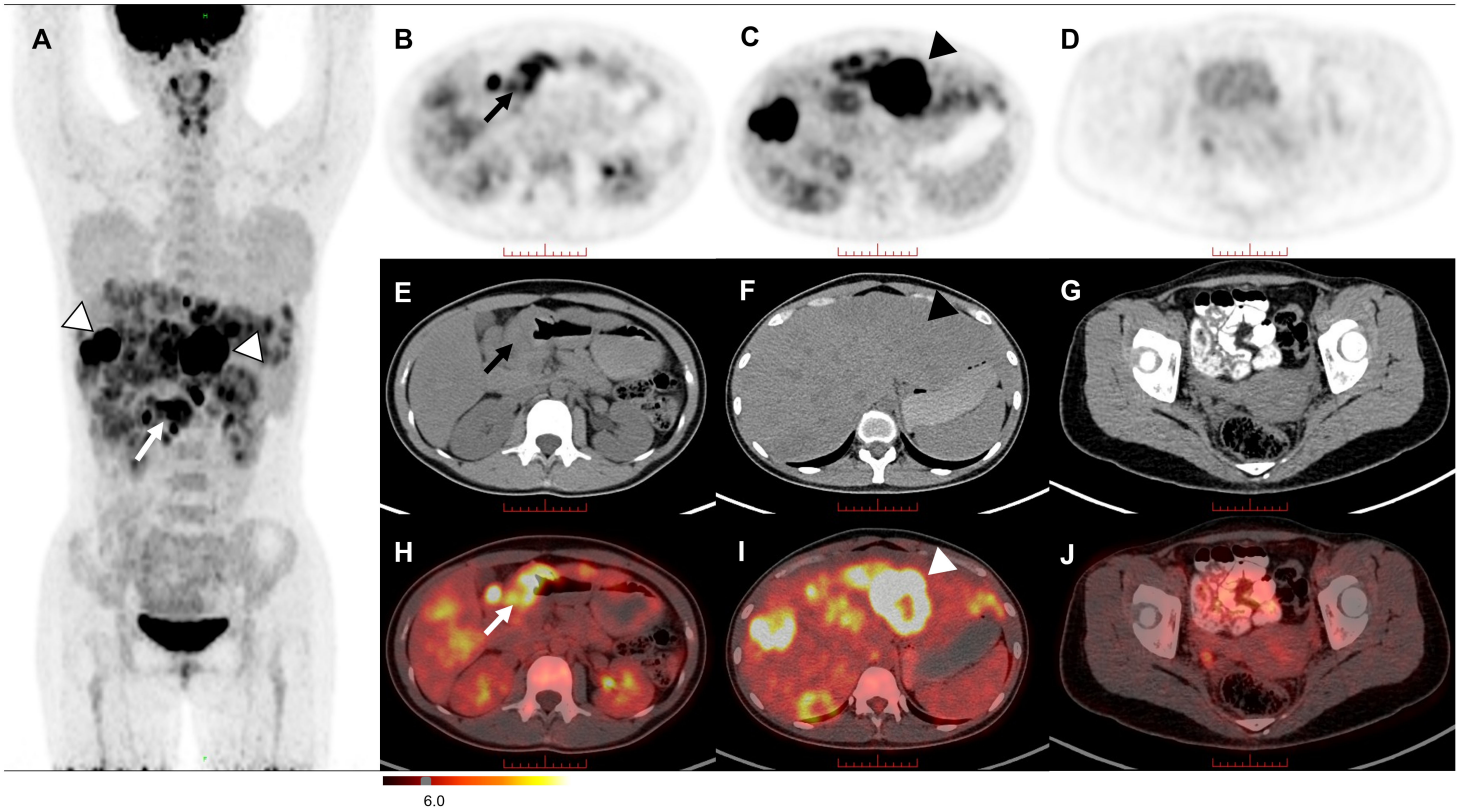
The MIP image (A) revealed intense uptake in the stomach (arrow) and liver (triangles). On axial views, remarkably ring-shaped high uptake was observed in the thickened gastric sinus wall [(B, E, H) arrow] and the left lobe of the liver [(C, F, I) triangle]. No significant FDG uptake was observed in the pelvic region (D, G, J).
